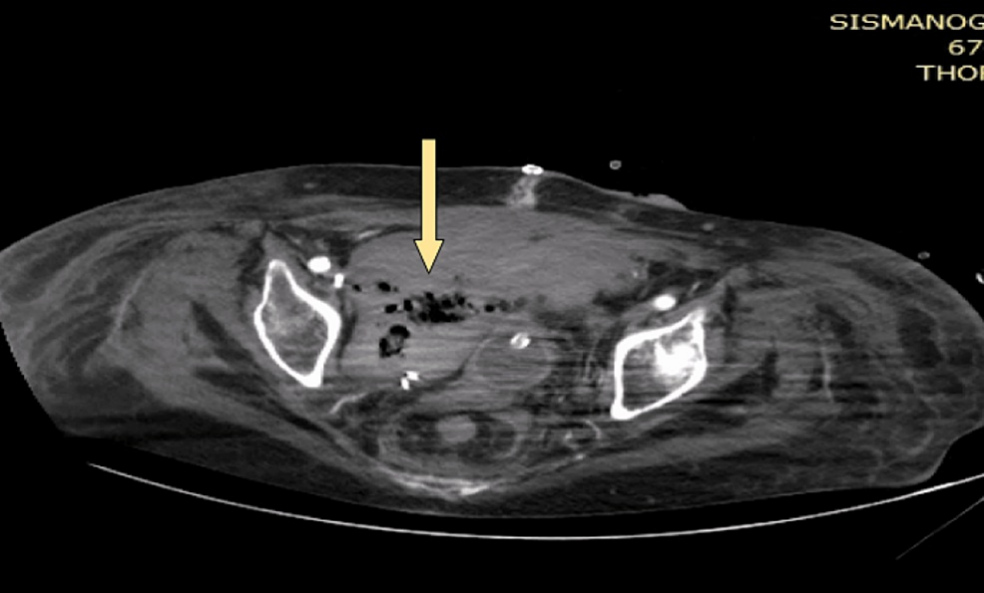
CT scan showing hematoma with air bubbles (axial) CT: computed tomography
